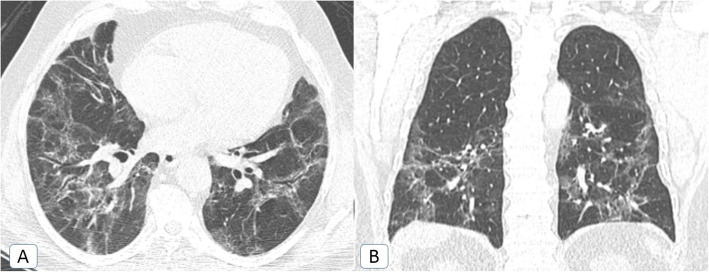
Post-COVID fibrosis: a 57-year-old male COVID-19 patient. Chest CT was done 3 months after the COVID-19 infection. A Axial MPR (lung window) and B coronal MPR (lung window), showing bilateral upper and lower lobar persistent ground-glass patches and irregular fibrotic changes with parenchymal distortion and mild traction bronchiectatic changes
